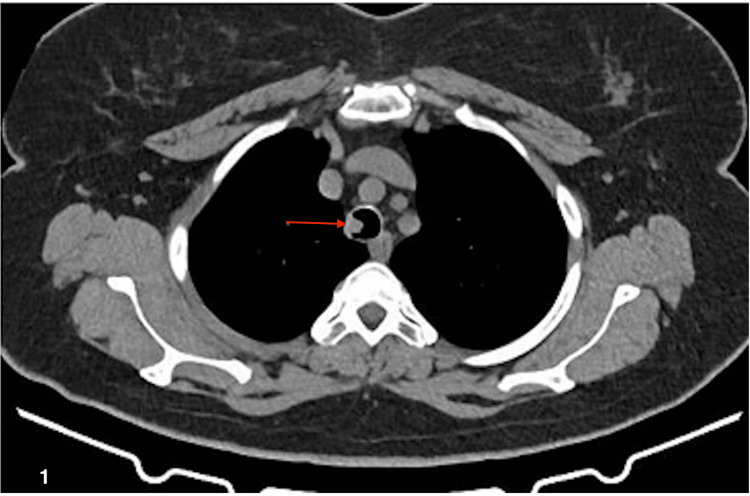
CT scan showing tracheal endoluminal lesion
